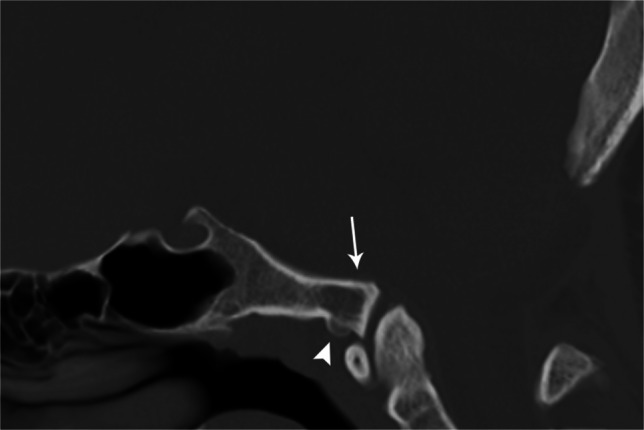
Bone window sagittal CT of the brain in a 13-year-old female demonstrates abnormal widening of the dens with an articular surface for the dens. In some cases, condylus tertius may project inferiorly from the midline basiocciput and may articulate with the anterior arch of C1 and/or the dens. Note the prominent clival tubercle (*arrowhead*), a remnant of the hypochordal bow of the proatlas
